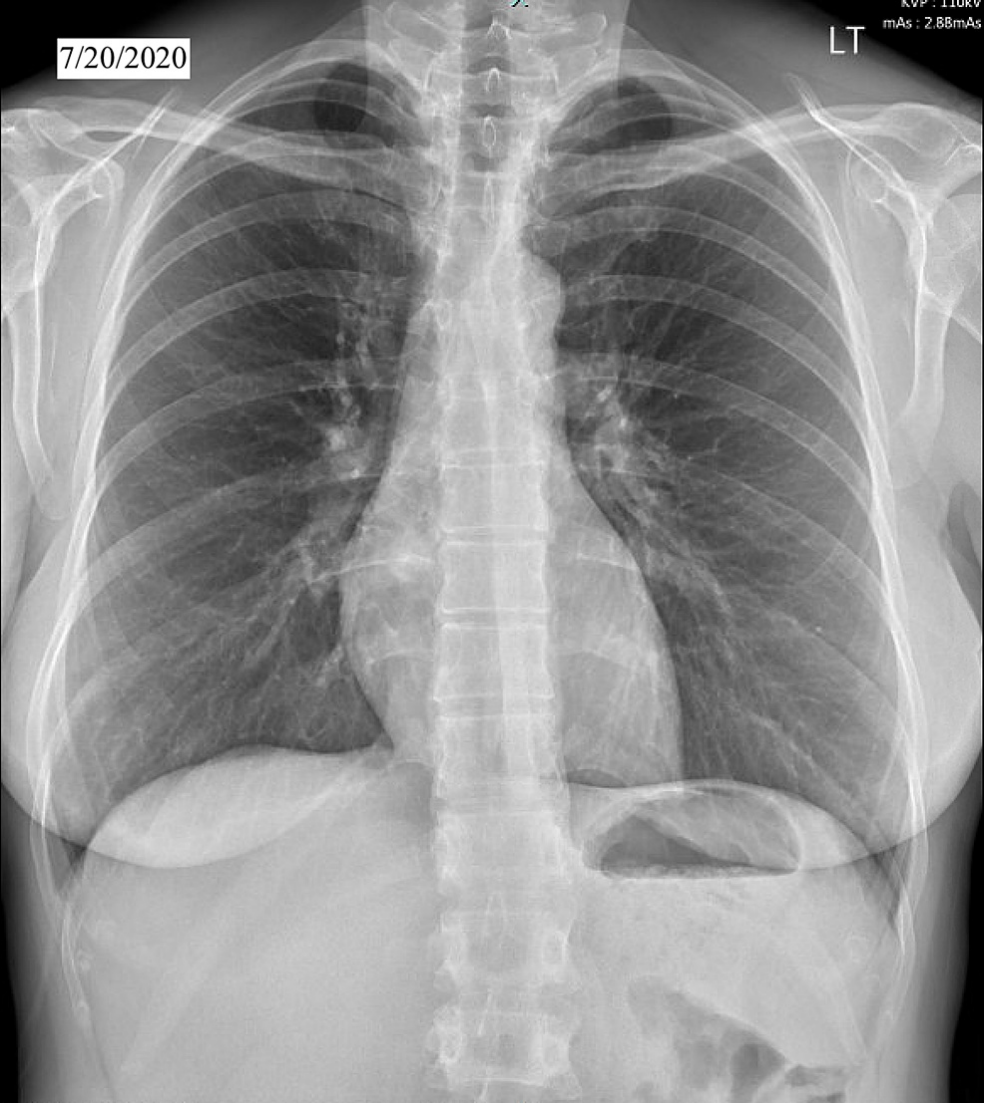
Chest X-ray from 7/20/2020. Normal chest X-ray.
